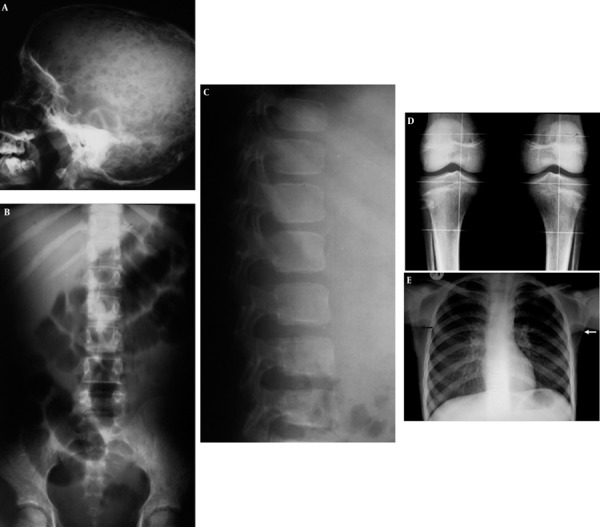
A 13-year-old girl with ALL presenting with pallor, fever, headache and bone pain A, Skull X-ray reveals multiple lytic lesions; B, Lumbosacral AP X-ray reveals permeative bony lesions in iliac bones with reduced vertebral height of L5; C, Lumbosacral lateral X-ray reveals reduced vertebral height of L5 with reduced bone density and lucent zone beneath the vertebral endplate; D, Knee X-ray reveals reduced bone density with permeative appearance; E, CXR reveals permeative bony lesions in bilateral scapular bones (arrows).
